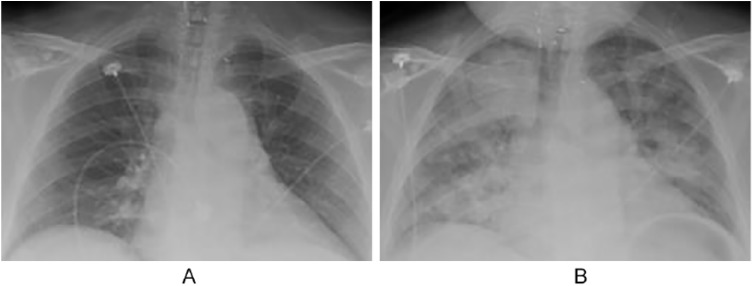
(A) Chest X-ray on admission. (B) Chest X-ray on hospital day 2 showing diffuse bilateral alveolar infiltrates.
